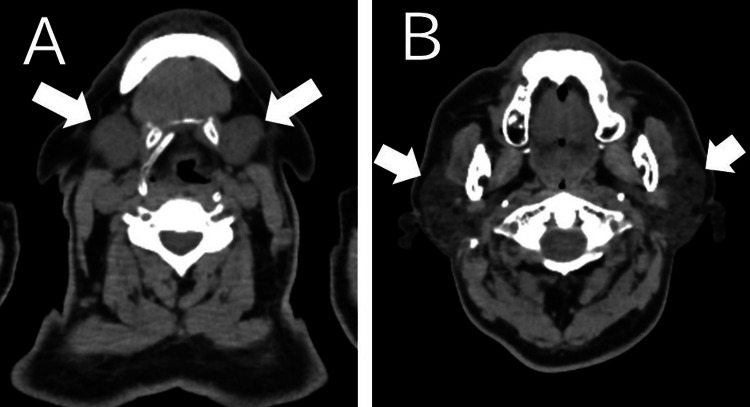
CT findings (HOD8). (A) Arrows indicate a reduction in bilateral submandibular gland size (44 × 25 mm). (B) Arrows indicate a reduction in bilateral parotid gland size (31 × 23 mm) CT: computed tomography, HOD8: hospital day 8
